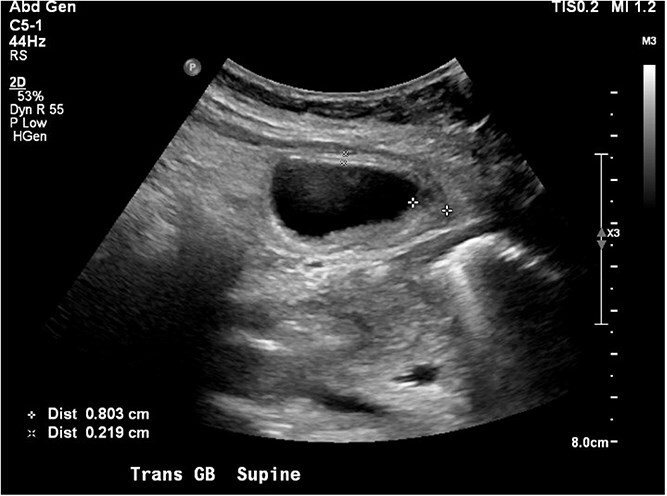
Ultrasound findings concerning for acute cholecystitis when the patient initially presented to the ED.
